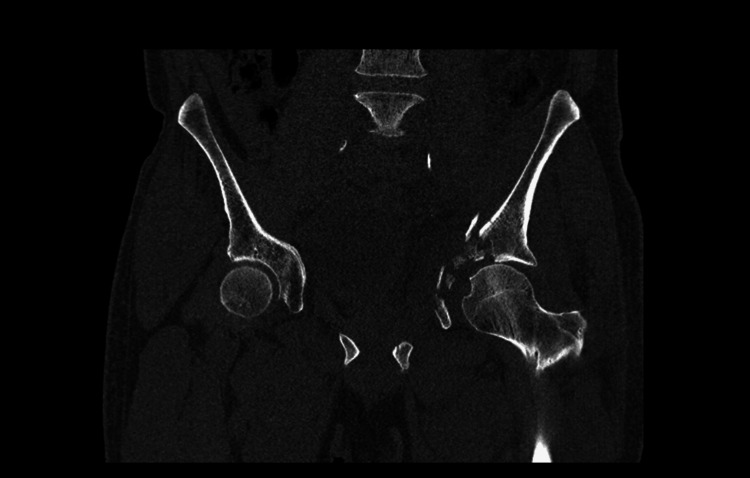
Coronal pelvic CT scan showing non-union of a left acetabular fracture A CT scan of the pelvis showed that the anterior column and wall had healed together, whereas the posterior column had not healed and showed medial migration and a distortion of the femoral head due to an impacted fracture
